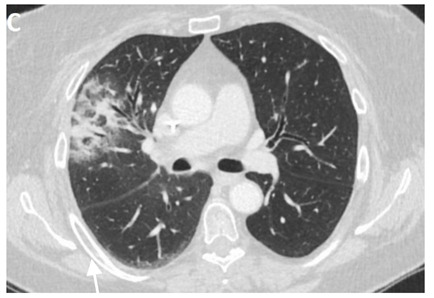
Post radiation therapy axial CT chest (image C), 1.5 months later showing ground-glass opacity and patchy airspace consolidation in RML conforming to the area of radiation field denoted by an arrow.
